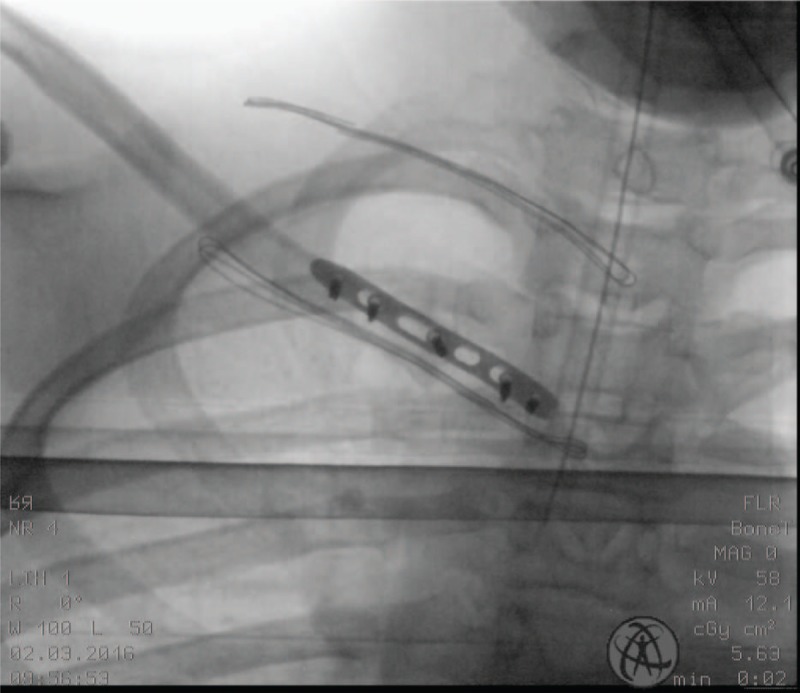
Postoperative imaging with plain radiographs showing correct realignment of the clavicle.
